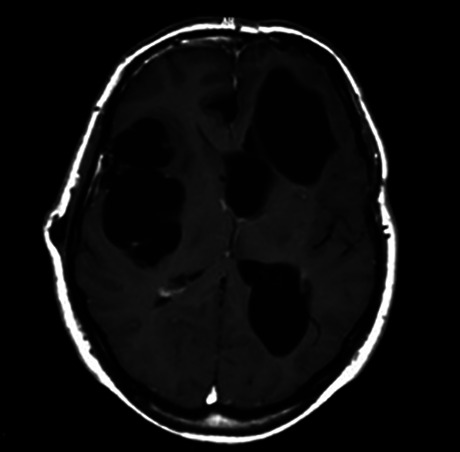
MRI multiple giant cysticerci.
